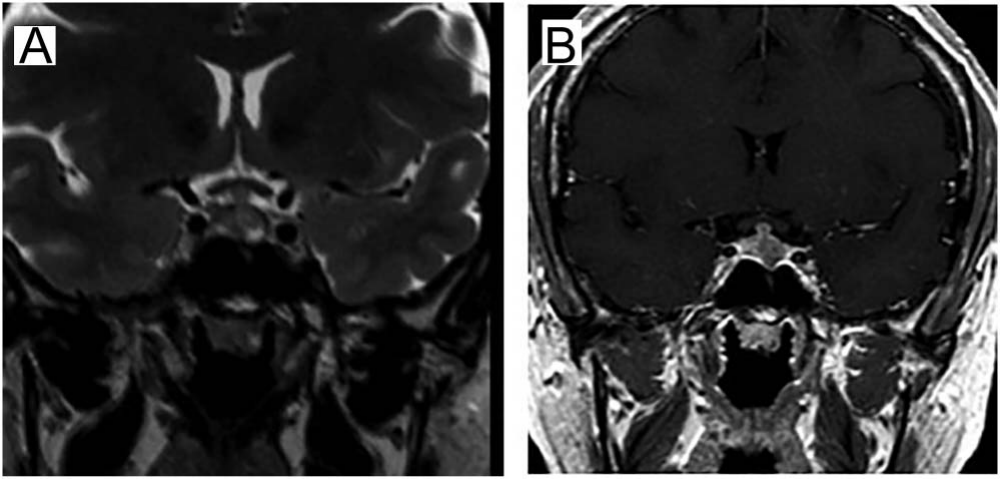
MRI of the sella turcica, coronal slices weighted in T2 (A) and post-contrast T1 (B), demonstrates an elongated formation measuring 1.0 × 0.5 cm, suggestive of a pituitary adenoma.
